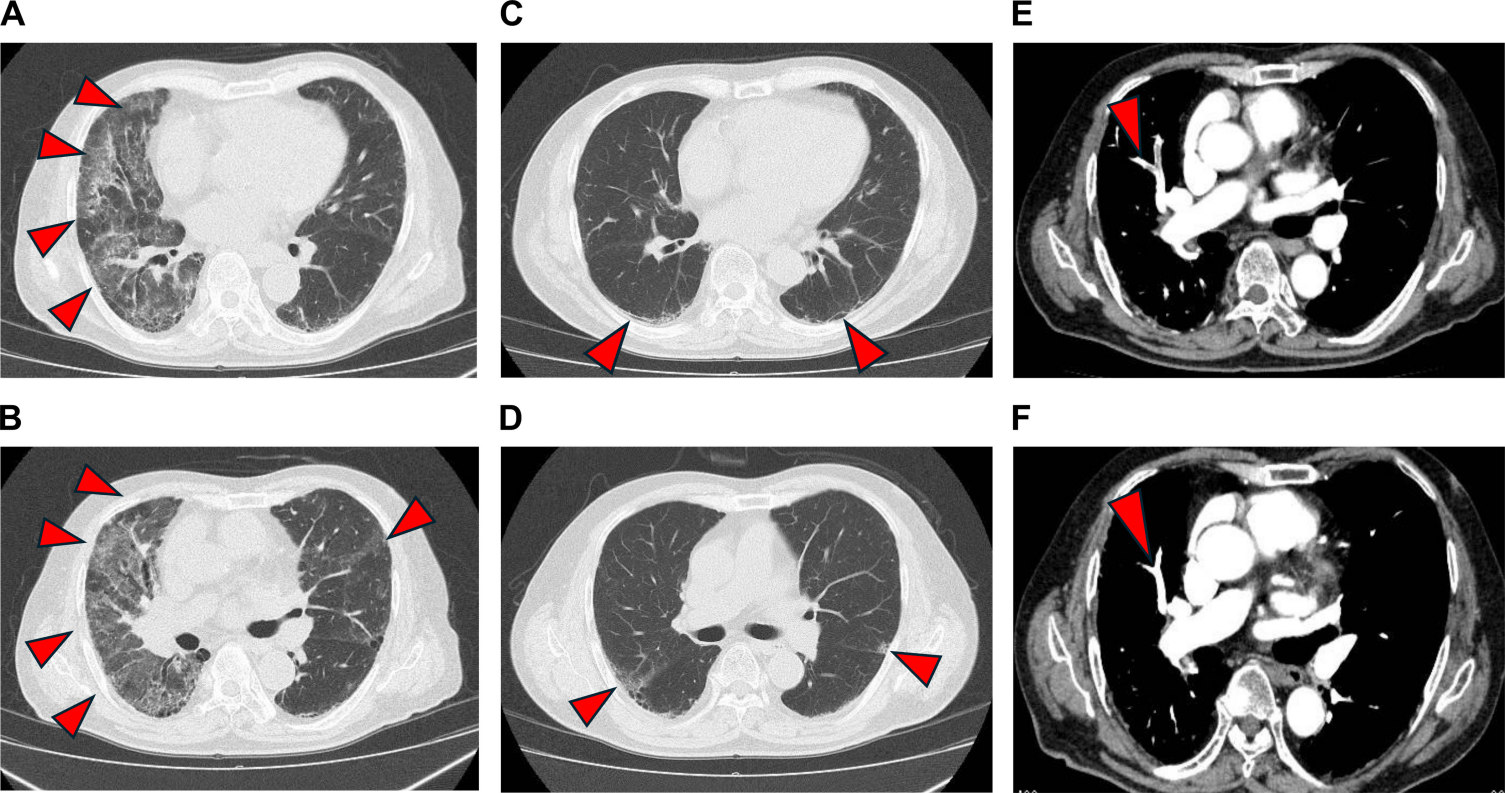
Chest CT scan showed ground‐glass opacities in the bilateral lung fields (A, B), which were not observed 6 years back (C, D). Ground‐glass opacities and reticular shadows in the bilateral peripheral lung field, suspected to be signs of chronic interstitial pneumonia, were observed (D, arrows). Contrast‐enhanced CT scan revealed multiple contrast‐enhanced defects mainly in the peripheral lower lobe branches of the bilateral pulmonary arteries (E, arrows). Contrast‐enhanced CT scan performed 1 month after discharge showed that the pulmonary thrombus had improved (F, arrows).
